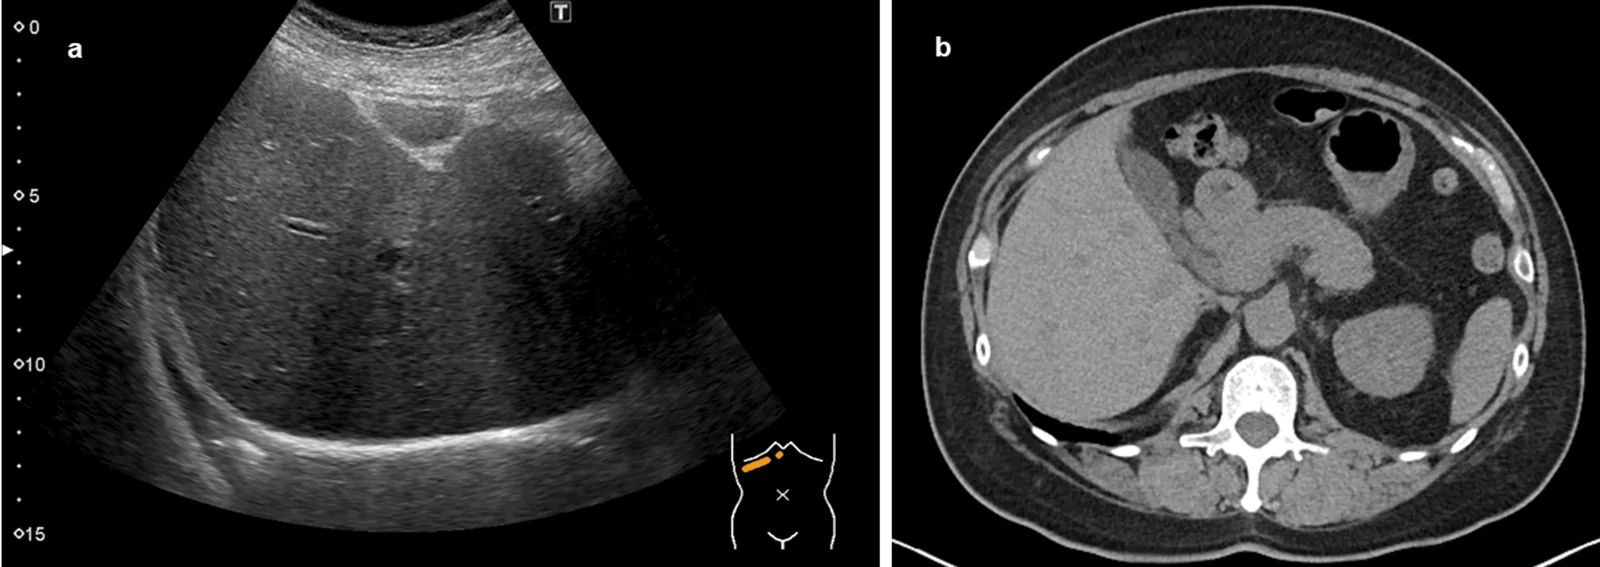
Abdominal images on the second admission. (a) Abdominal ultrasonography shows a smooth liver surface and patent perihepatic spaces without abnormal fluid collection. (b) Contrast-enhanced computed tomography shows no detectable intrahepatic or extrahepatic biliary tract obstruction, structural stricture, or space-occupying lesions.
